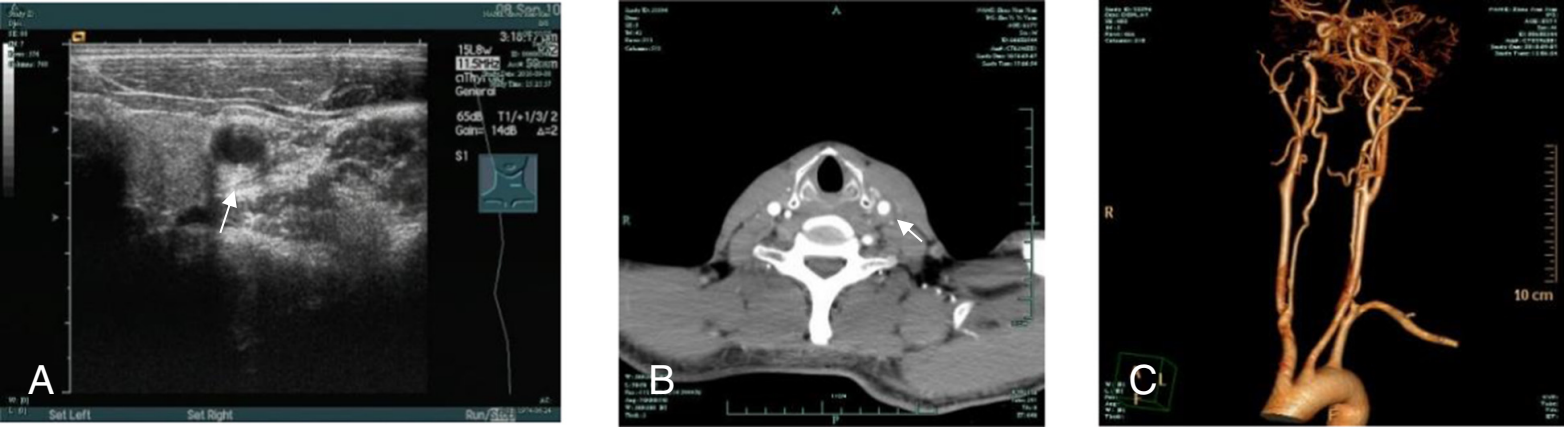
The BUS (A) and three-dimensional CT angiography (CTA) (B, C) revealed a mass wrapping around the segment of the vessel from left common carotid artery to internal carotid in the left neck (carotid body tumor suspected).
